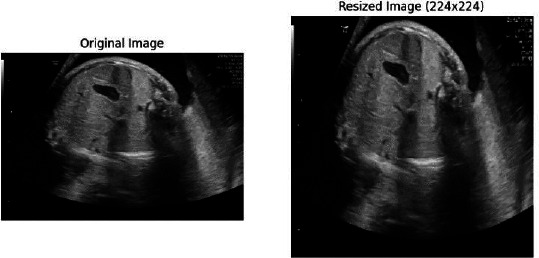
Comparison of original and resized ultrasound images (224x224).
